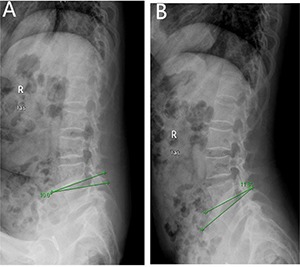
Angular motion of a 48 years old man,diagnosed with discogenic low back pain (LBP) for 2 years (A) delineates flexion angle measured by X-ray (L4-5). (B) indicates the extension angle measured by X-ray (L4-5).
